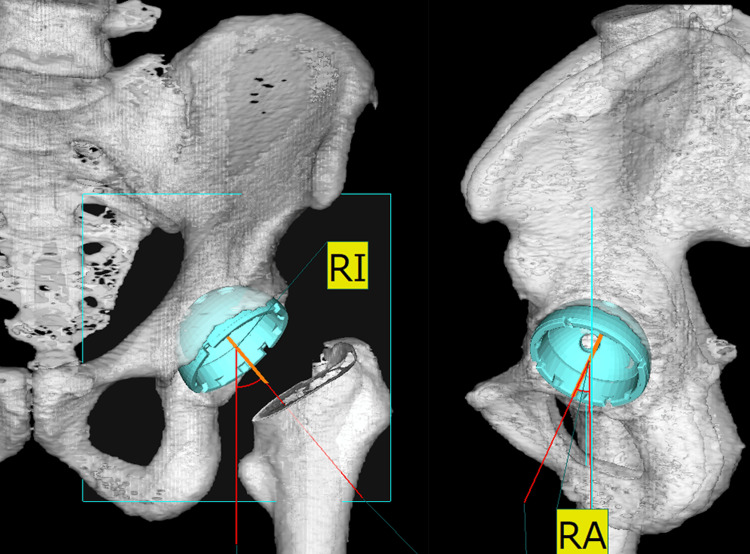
Radiographic visualization of acetabular component orientation The left image depicts the acetabular component orientation, with the RI measured to evaluate the angle of the cup's tilt in the coronal plane. The right image displays the RA, assessing the cup's rotation angle in the axial plane. RA, radiographic anteversion; RI, radiographic inclination
